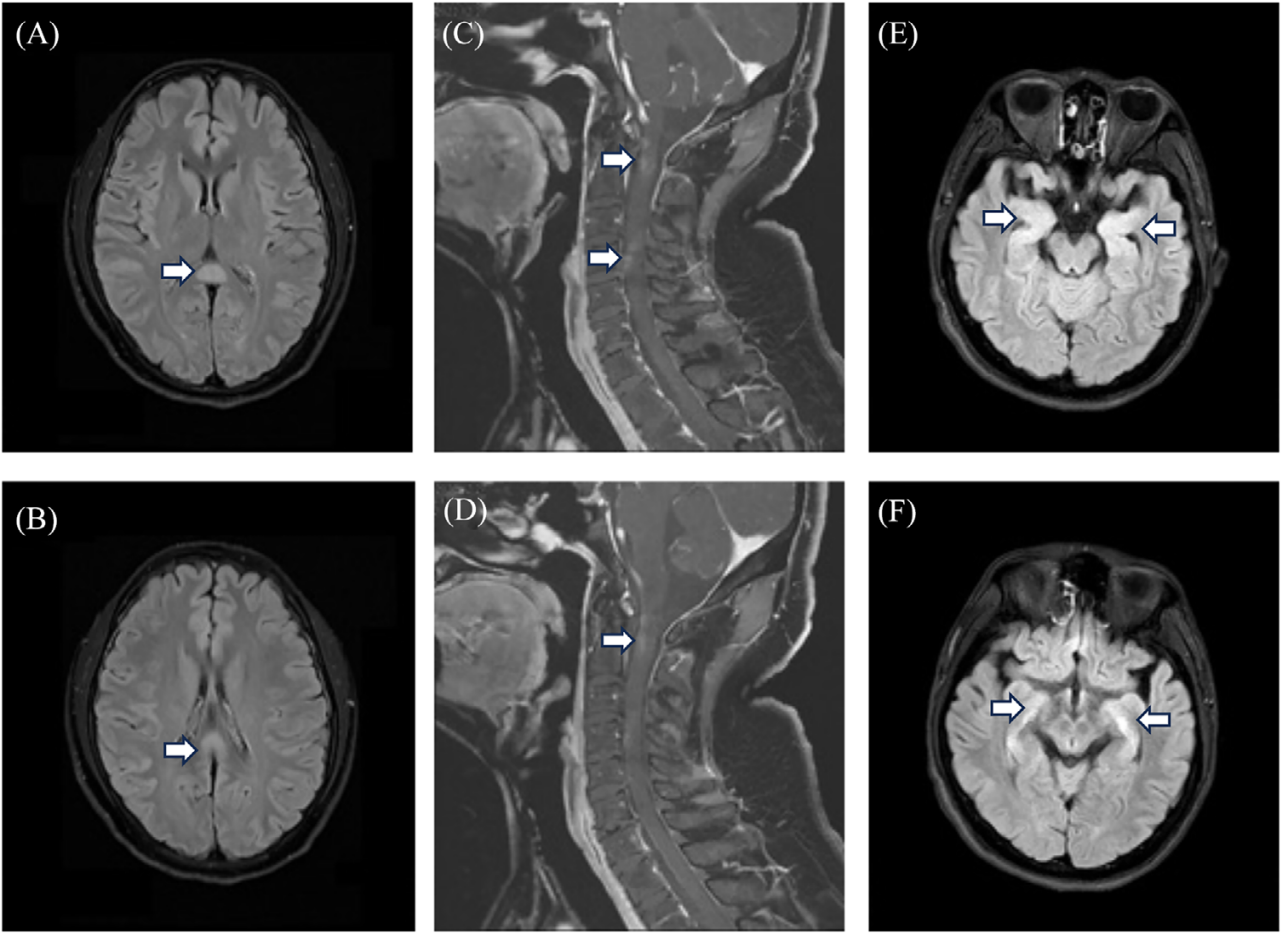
Images of brain MRI scans of Neuro‐COVID patients. (A and B) A 20‐year‐old woman presented with gradually worsening delirium after an upper respiratory tract infection. MR imaging of the brain demonstrated prominent T2‐FLAIR abnormalities in the splenium of the corpus callosum (Arrowheads). (C and D) A 55‐year‐old man with recurrent acute myelitis presented with progressively worsening numbness and weakness in the extremities. Contrast‐enhanced MRI of the cervical and thoracic spine exhibited abnormal enhancement of the cervical spinal cord and enhancement of the cervicothoracic meninges (Arrowheads). (E and F) A 19‐year‐old man presented with psychosis and behavior disorder. MRI of the brain prominent T2‐FLAIR abnormalities in the bilateral hippocampus (Arrowheads).
